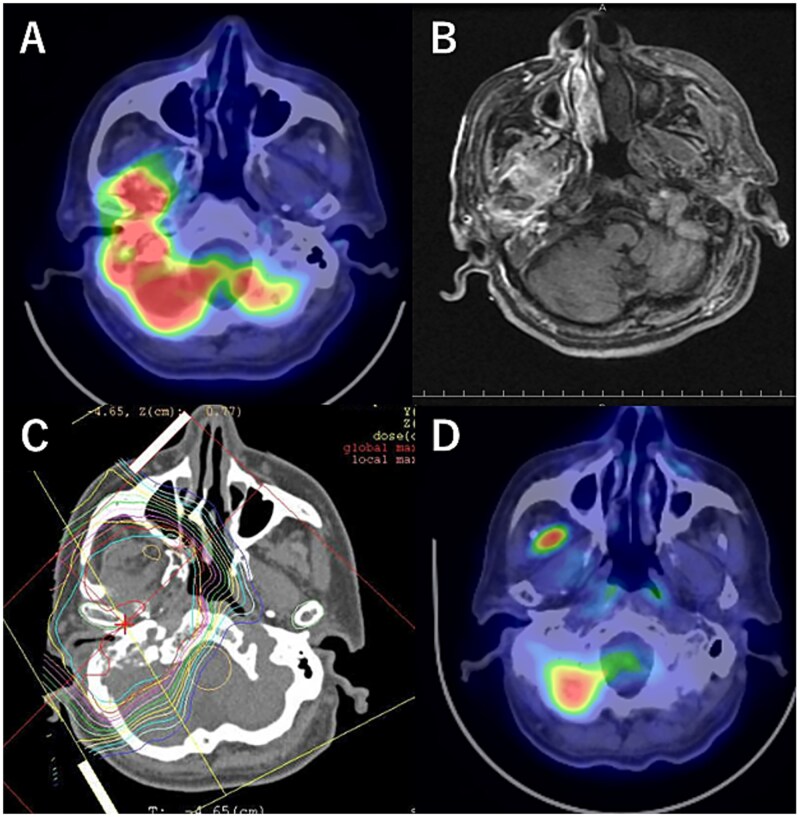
Representative case of a T4bN0M0 MEC treated with proton beam therapy (PBT) and intra-arterial infusion chemotherapy via superficial temporal artery (STA). (A) Image of fluorodeoxyglucose-positron emission tomography (18F-FDG-PET/CT) before PBT. (B) Flow check magnetic resonance imaging showed the entire tumour was enchanced. (C) Dose distribution of PBT planning. (D) PET/CT performed 1 year after PBT showed that the FDG uptake of tumour was disappeared. The FDG uptake in masticator space reflected inflammation.
